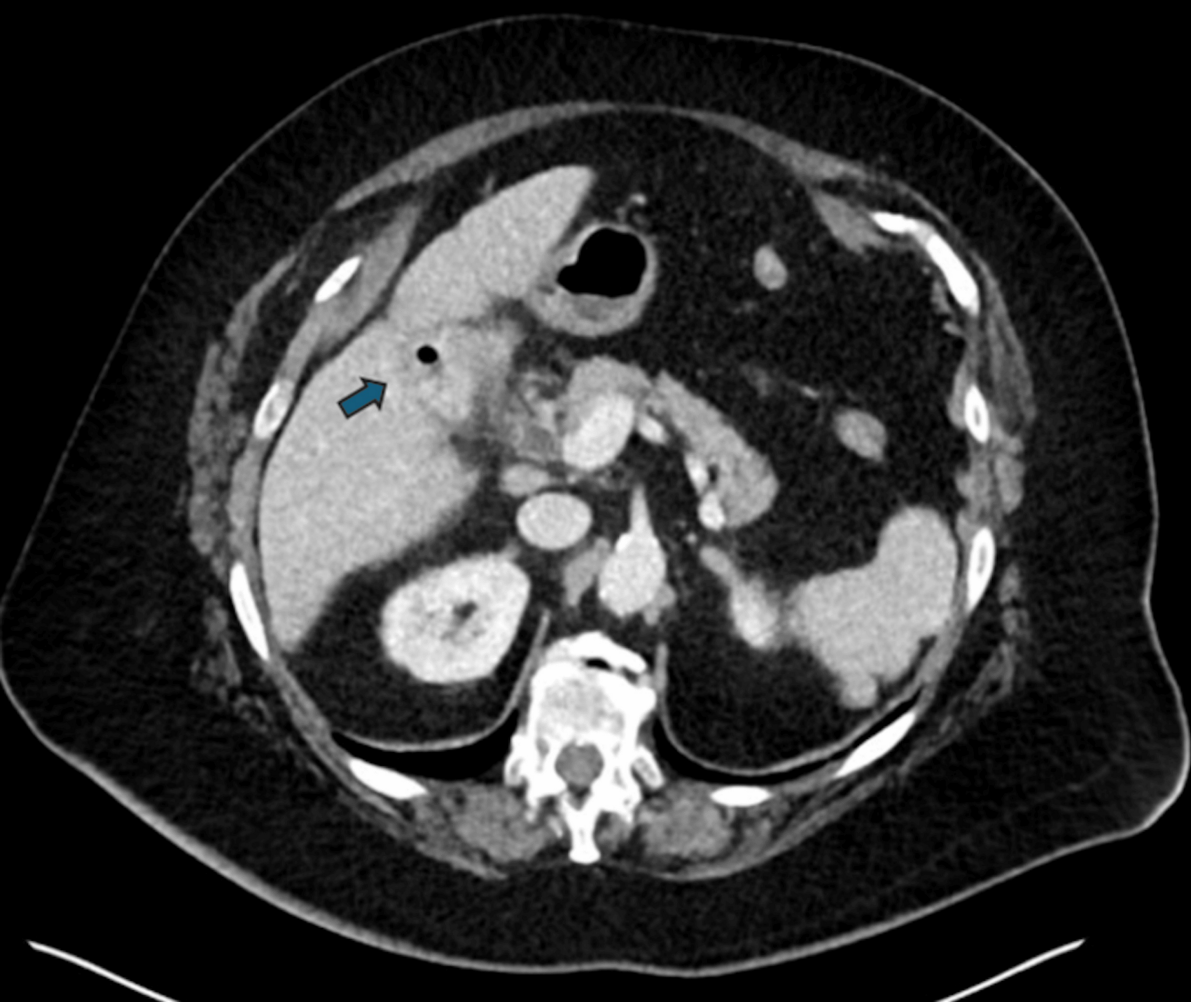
Pneumobilia in the gallbladder This CT image of the upper abdomen focuses on the gallbladder and biliary system. The gallbladder (blue arrow) shows the presence of air, known as pneumobilia, which appears as dark areas within the biliary tree.
